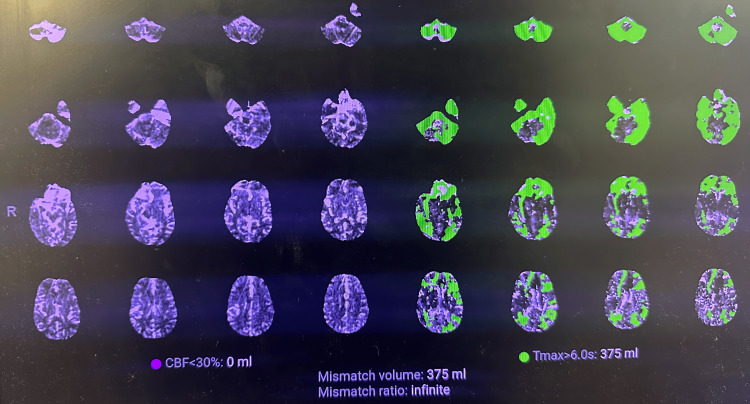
CT perfusion imaging demonstrating large perfusion mismatch in posterior circulation stroke. Multimodal CT perfusion maps demonstrate a large region of delayed perfusion (Tmax >6 seconds, green) involving bilateral cerebral and cerebellar hemispheres (arrows), without a corresponding reduction in cerebral blood flow (CBF <30%), indicating absence of a defined infarct core. This mismatch profile reflects a substantial volume of hypoperfused yet potentially salvageable tissue, supporting a physiology-driven approach to reperfusion therapy.
